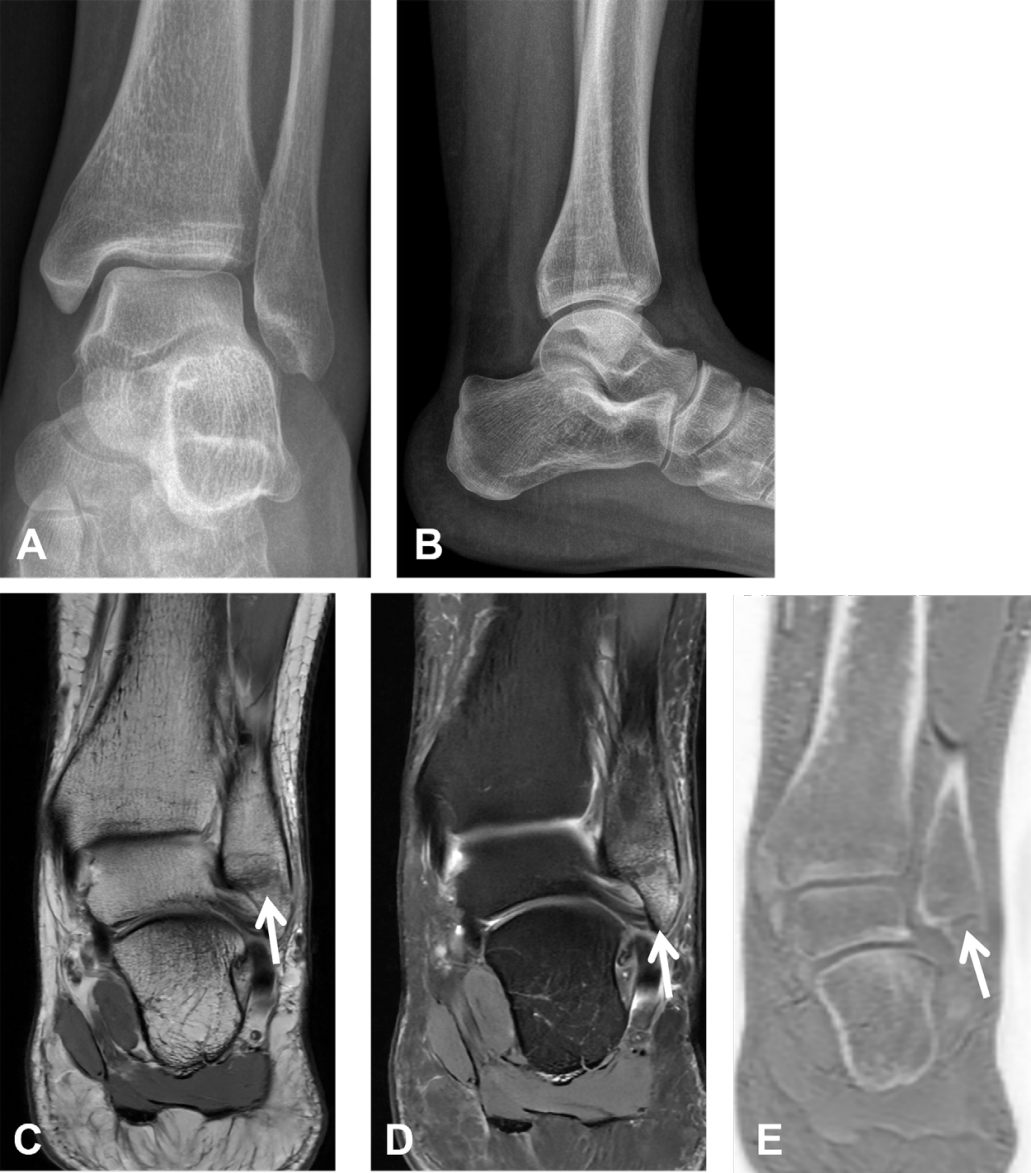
A distal fibular fracture in a 45-year-old female patient following an ankle sprain. (A, B) Anteroposterior and lateral ankle radiographs show no definite fracture. (C, D) Coronal proton density and coronal fat-suppressed T2-weighted MR images demonstrate a subtle hypointense line in the distal fibula with associated bone marrow edema (white arrows). (E) The coronal ZTE image clearly depicts the cortical fracture line.
